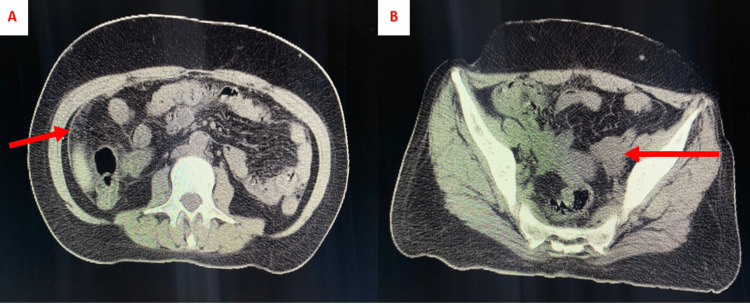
CT abdominopelvic sections of the patient (A) Thickening of the peritoneal sheets in favor of carcinomatosis (red arrow). (B) Enlarged left ovary with the presence of a solid-cystic mass of 3 cm (red arrow).
